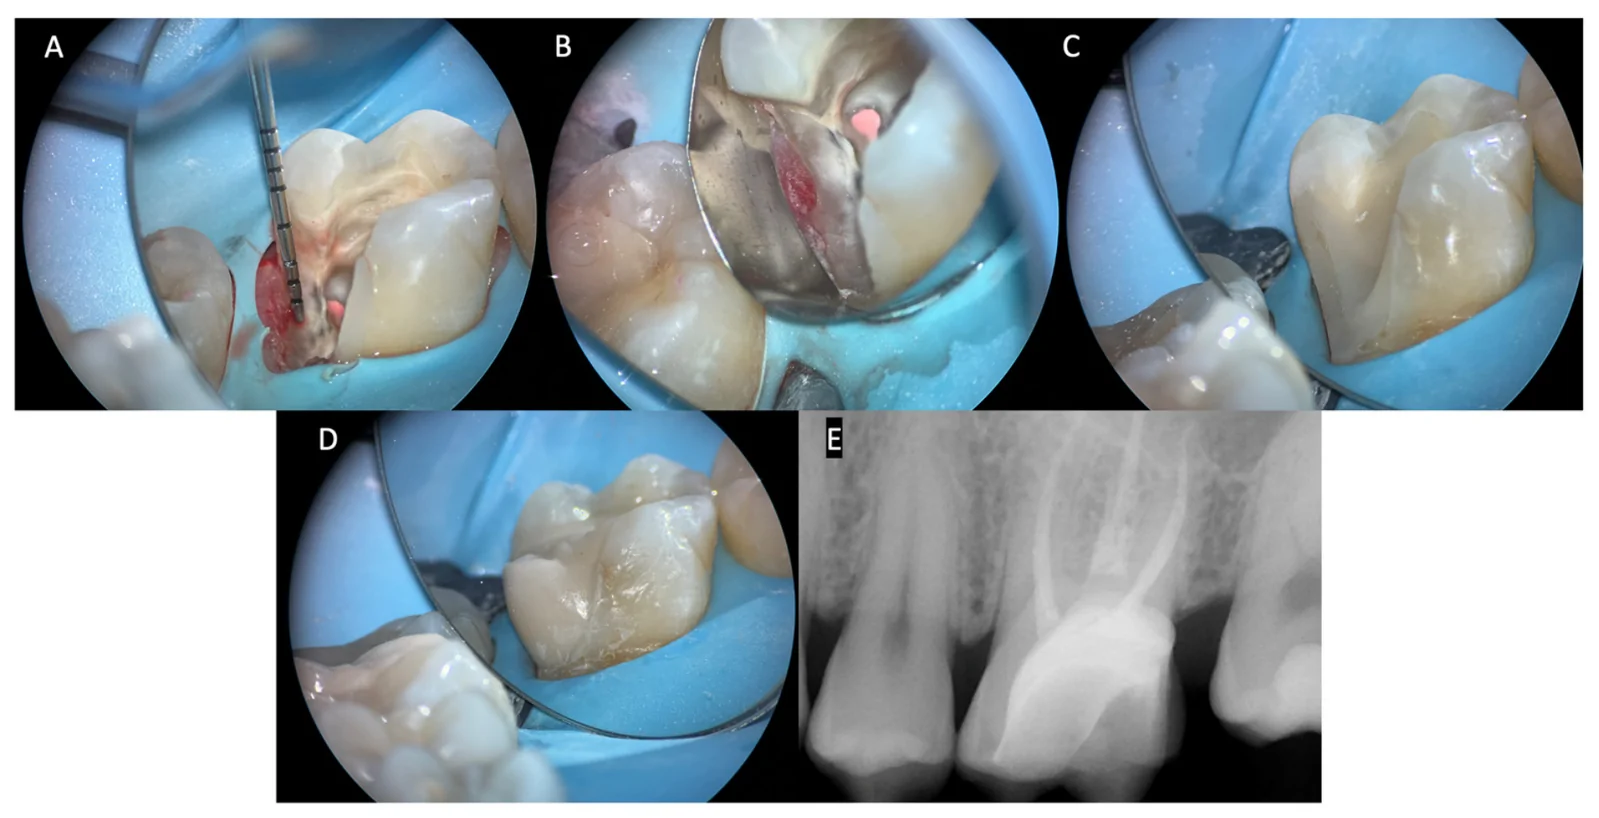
Zone 3 clinical scenario. (A) Initial lateral view with evaluation of margin depth using a periodontal probe; (B) lateral view of matrix placement; (C) completion of deep margin elevation; (D) final restoration; and (E) final radiograph.
